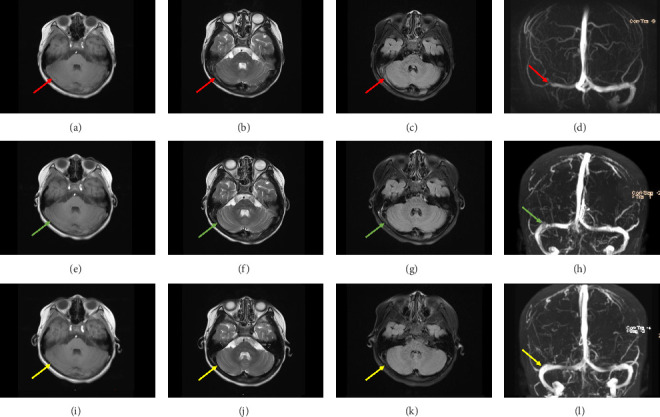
Dynamic changes in MRI/MRV images of patients before and after treatment. Pretreatment (a–d): (a) Axial T1-weighted image (T1WI) showing mixed signals, predominantly isointense and hyperintense, in the right transverse sinus (red arrow). (b) Axial T2-weighted image (T2WI) showing high signal intensity in the right transverse sinus (red arrow). (c) Axial T2 FLAIR image showing high signal intensity in the right transverse sinus (red arrow). (d) Coronal MRV image showing thrombosis in the right transverse sinus and sigmoid sinus, with loss of flow void (red arrow). 7 Days posttreatment (e–h): (e) Axial T1WI showing mixed signals, predominantly isointense and hyperintense, in the right transverse sinus (green arrow). (f) Axial T2WI showing partial recanalization of the right transverse sinus, with T2WI returning to low signal intensity (green arrow). (g) Axial T2 FLAIR image showing partial recanalization of the right transverse sinus, with FLAIR returning to low signal intensity (green arrow). (h) Coronal MRV image showing partial restoration of flow void in the right transverse sinus and sigmoid sinus (green arrow). 3 Months Posttreatment (i–l): (i) Axial T1WI showing no significant abnormal signal in the right transverse sinus (yellow arrow). (j) Axial T2WI showing patency of the right transverse sinus, with low signal intensity (yellow arrow). (k) Axial T2 FLAIR image showing patency of the right transverse sinus, with low signal intensity (yellow arrow). (l) Coronal MRV image showing normal flow void in the right transverse sinus and sigmoid sinus (yellow arrow).
